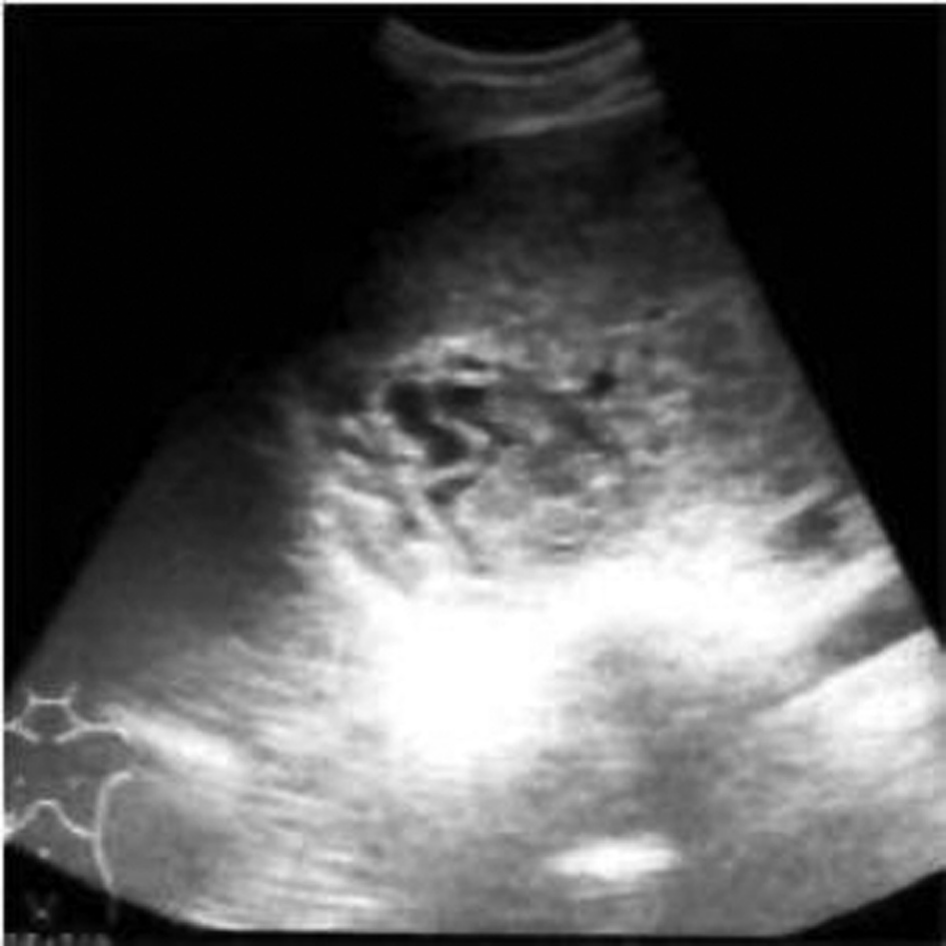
Figure 6. Follow up sonography at three months showing pseudotumor appearance
